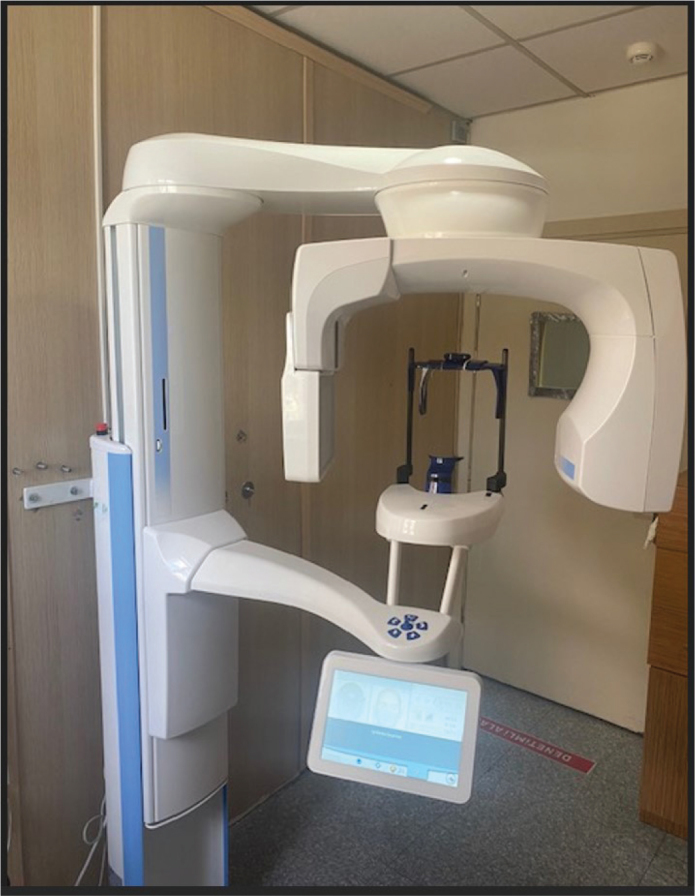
Cone-beam computed tomography device used for image acquisition (Planmeca ProMax 3D Mid).
